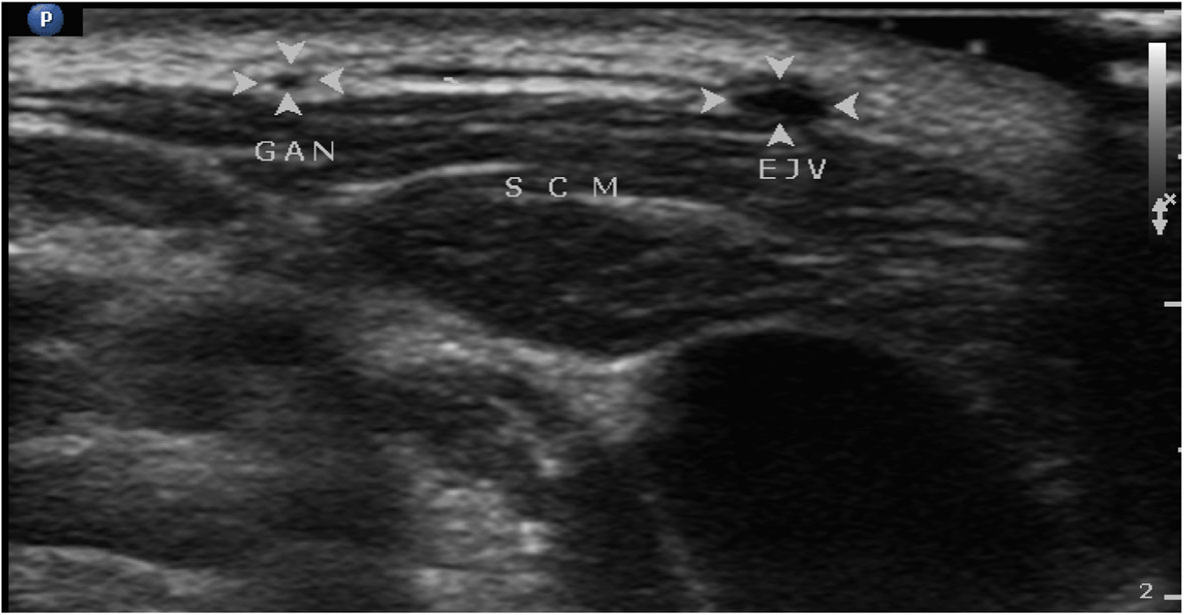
Cross sectional ultrasound view of the great auricular nerve (GAN, indicated by the arrows) at the surface of sternocleidomastoid muscle (SCM). EJV: external jugular vein. Right side = medial
